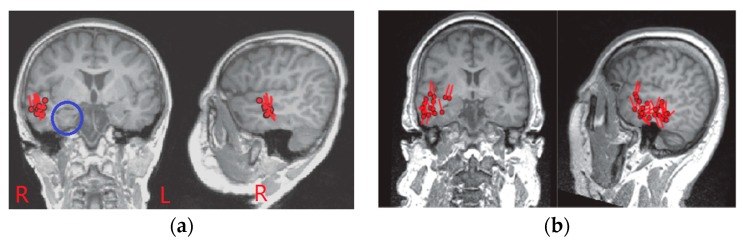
Magnetic source imaging results based on the SPS method based on all spikes. (a) Localization results for the first patient displayed on individual MRI from coronal and sagittal views, wherein the hippocampal region was denoted by a blue circle on coronal view, and the red mark “R” (or “L”) denoted the right (or left) side of the brain; (b) Localization results for the second patient displayed on individual MRI from coronal and sagittal views.
